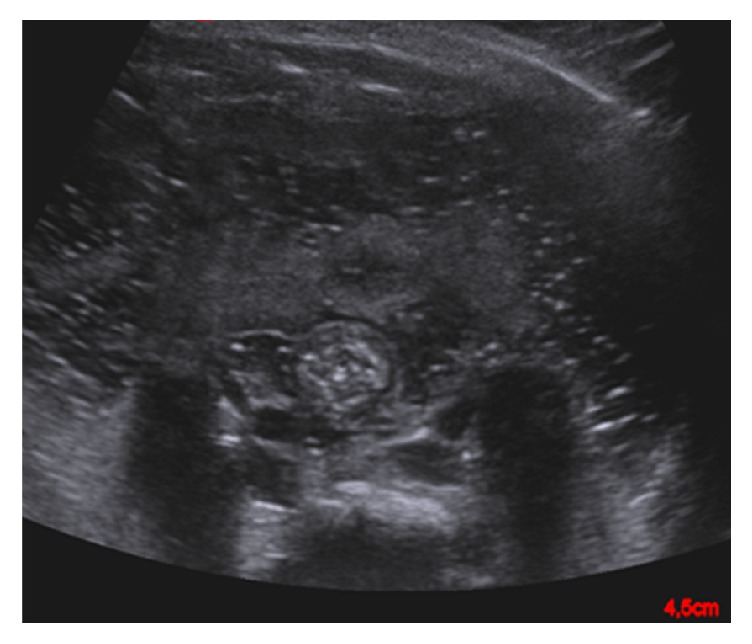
Ultrasound performed on the first day of life revealed free intraabdominal fluid.
